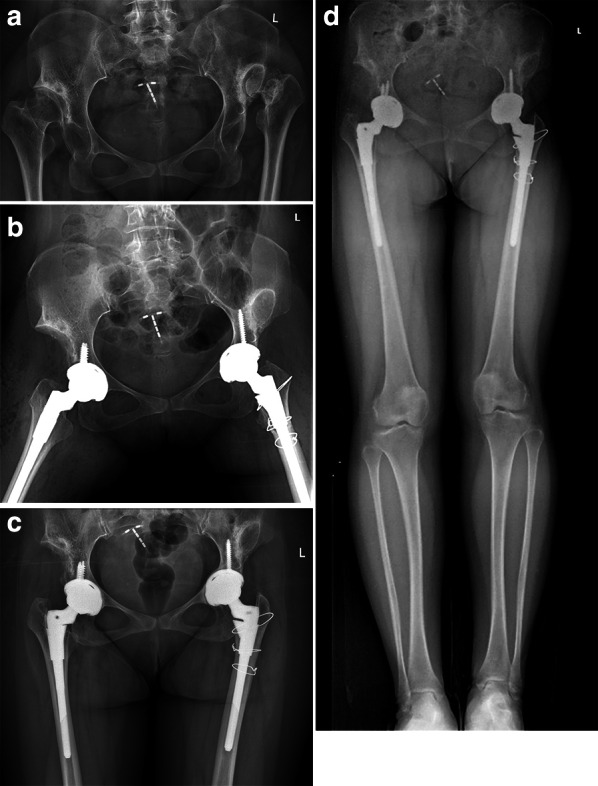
Preoperative anterior–posterior X-ray showing a bilateral DDH (right hip as Crowe III and left hip as Crowe IV) in a 51-year-old female patient (a). Postoperative X-ray showing bilateral anatomical reconstruction (b). SSTO was performed in the Crowe IV hip, and the osteotomy length was 2.0 cm. At final follow-up, radiographic evaluation after 1.8 years showing no loosening (c). Full-length standing anteroposterior radiograph showing that the right leg was 1.3 cm longer than the left leg (d)
